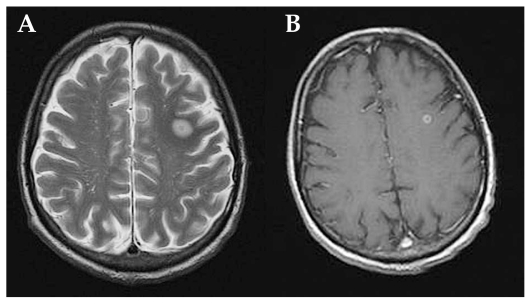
AIDS-related neuro-toxoplasmosis: single toxoplasmic lesion in the left frontal lobe (T2-weighted sequence, A), which shows ring-shaped enhancement after contrast injection (T1-weighted sequence, B).
